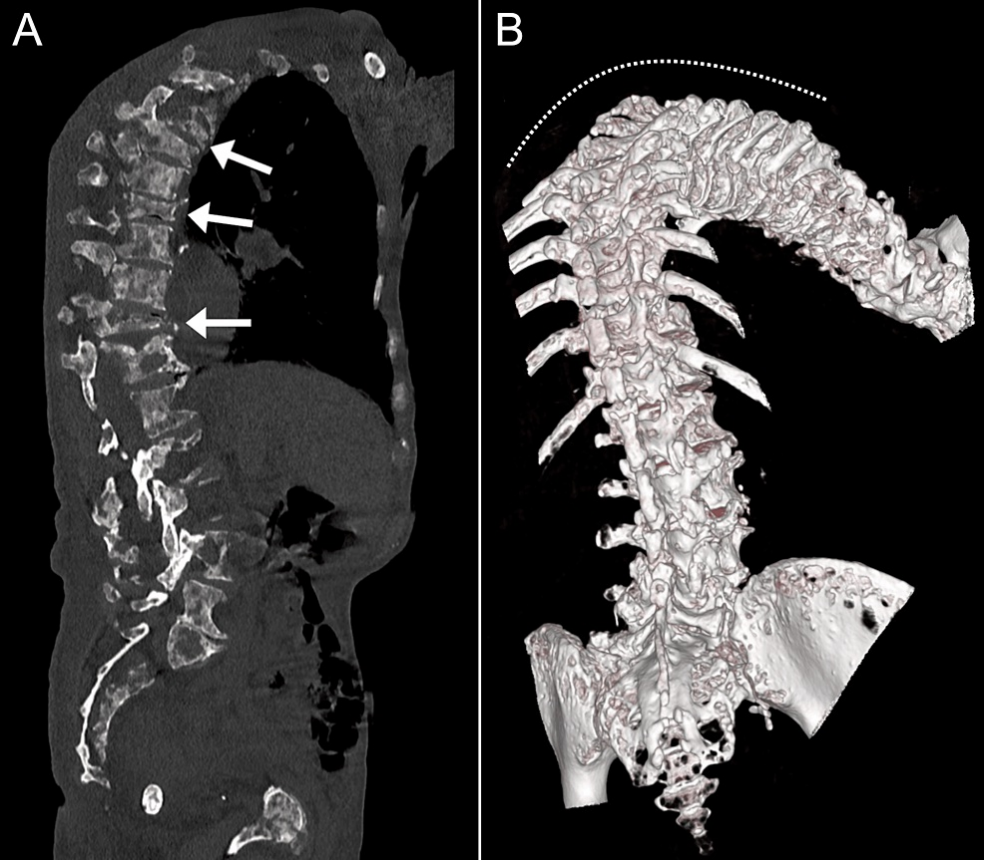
Sagittal (A) and three-dimensional reconstruction (B) of computed tomography (CT). This imaging revealed widespread mixed lytic/sclerotic changes, pathological anterior wedge compression fractures of the T5, T8, and T11 vertebral bodies (arrows), causing severe thoracic spine kyphoscoliosis (dotted line), several rib fractures (not indicated), and a grade 1 L2/L3 and L4/L5 spondylolisthesis (not indicated).
